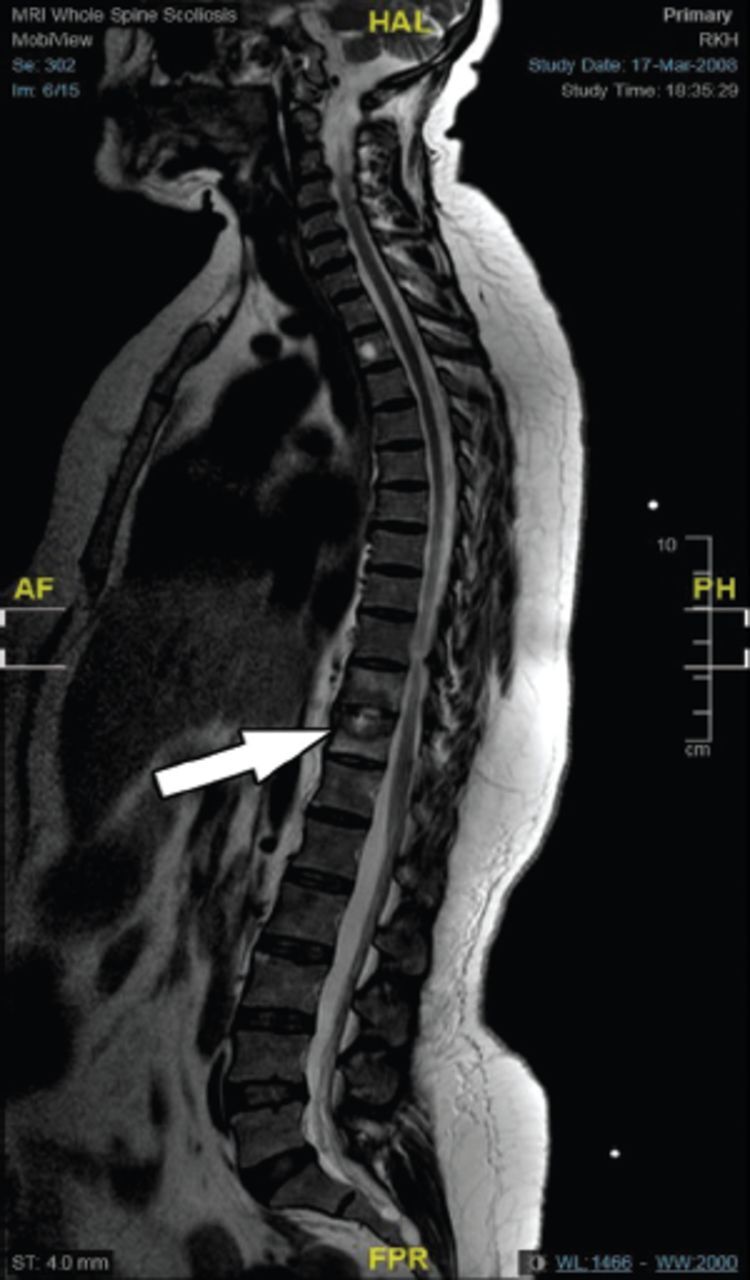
Magnetic resonance image of the spine. D10 and D11 vertebral bodies show low signal intensity on T1 with high sginal intensity on T2 weighted image with heterogenous enhacnces on post contrast evidence of metastatic disease.
